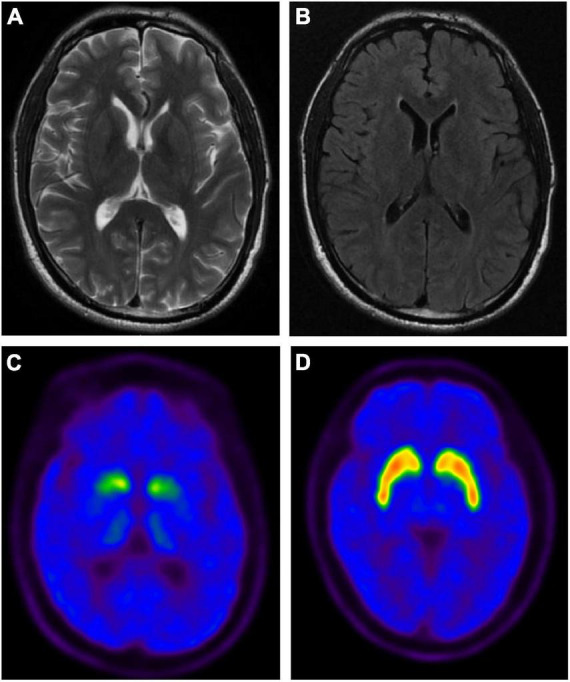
The brain magnetic resonance imaging (MRI) and positron emission tomography (PET) imaging of PD-1 patient with the premutation of *FMR1* gene CGG repeat expansion. Representative axial T2-weighted (A) and FLAIR (B) MRI images of PD-1. No abnormal signal intensities or atrophic changes are observed. Representative axial PET images of ^11^C-2β-carbomethoxy-3β-(4-fluorophenyl) tropane (^11^C-CFT) uptake in PD-1 (C) and healthy control (HC) (D), showing the distribution of dopamine transporters in PD-1 was significantly reduced in the bilateral putamen and caudate nuclei.
